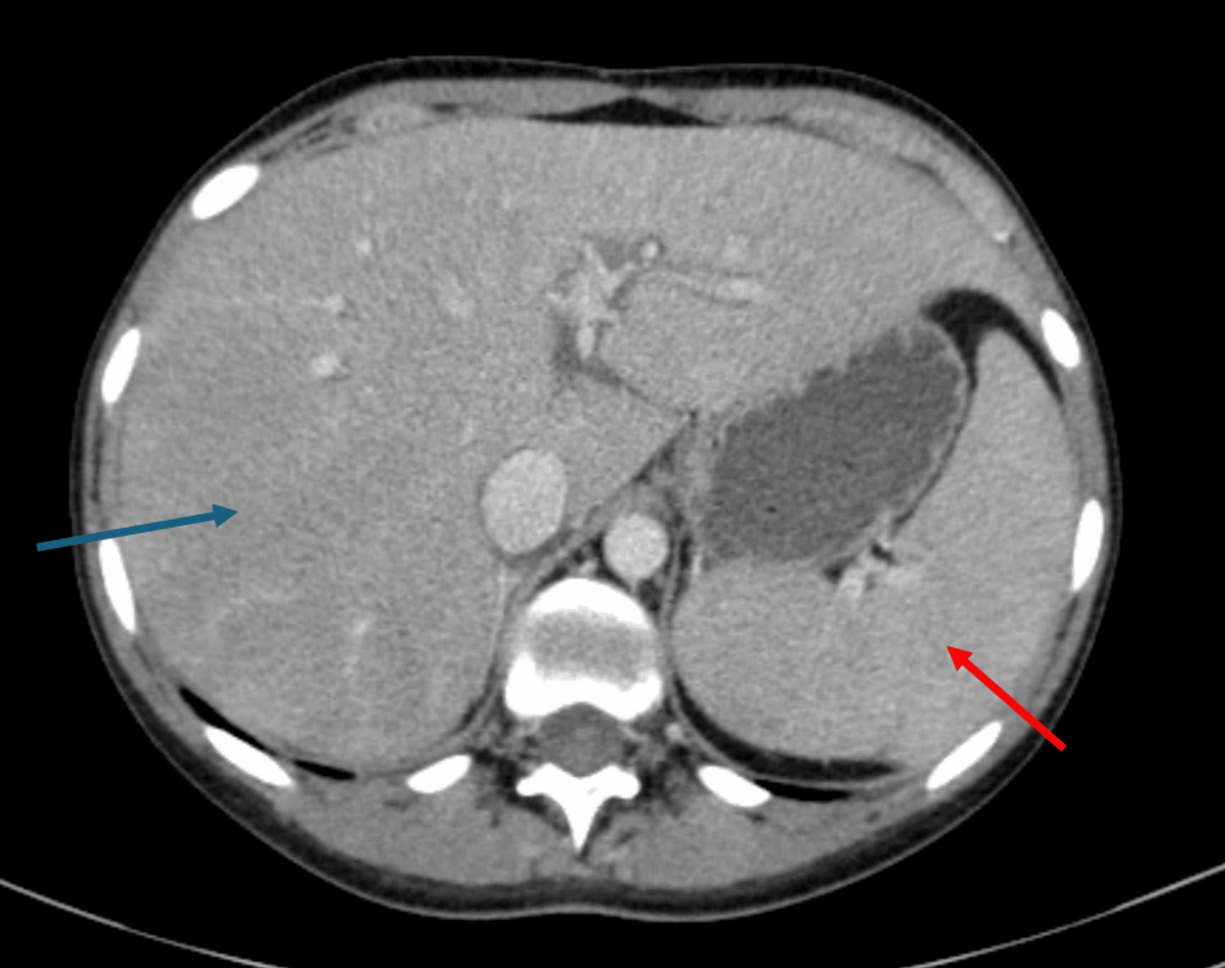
CT showing heterogenous hepatomegaly, 21.2 cm (blue arrow), and homogeneous splenomegaly, 13.3 cm (red arrow).
